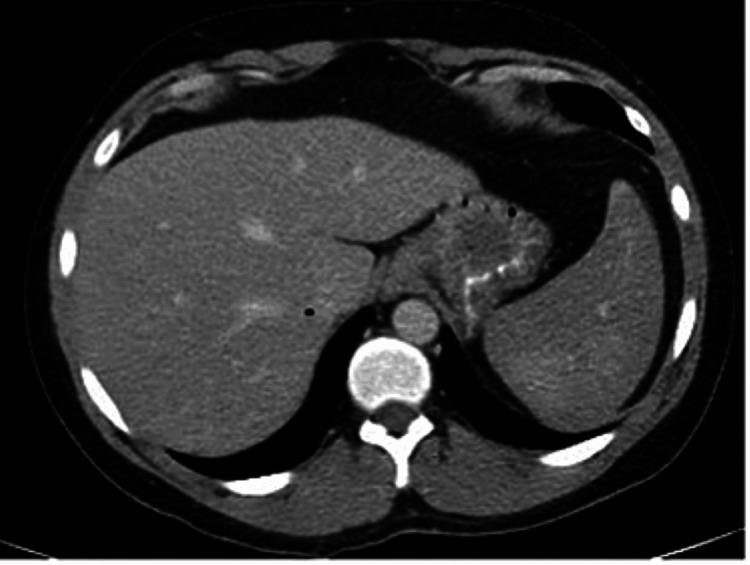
Initial computed tomography of the abdomen and pelvis showing splenic enhancement on day one.
